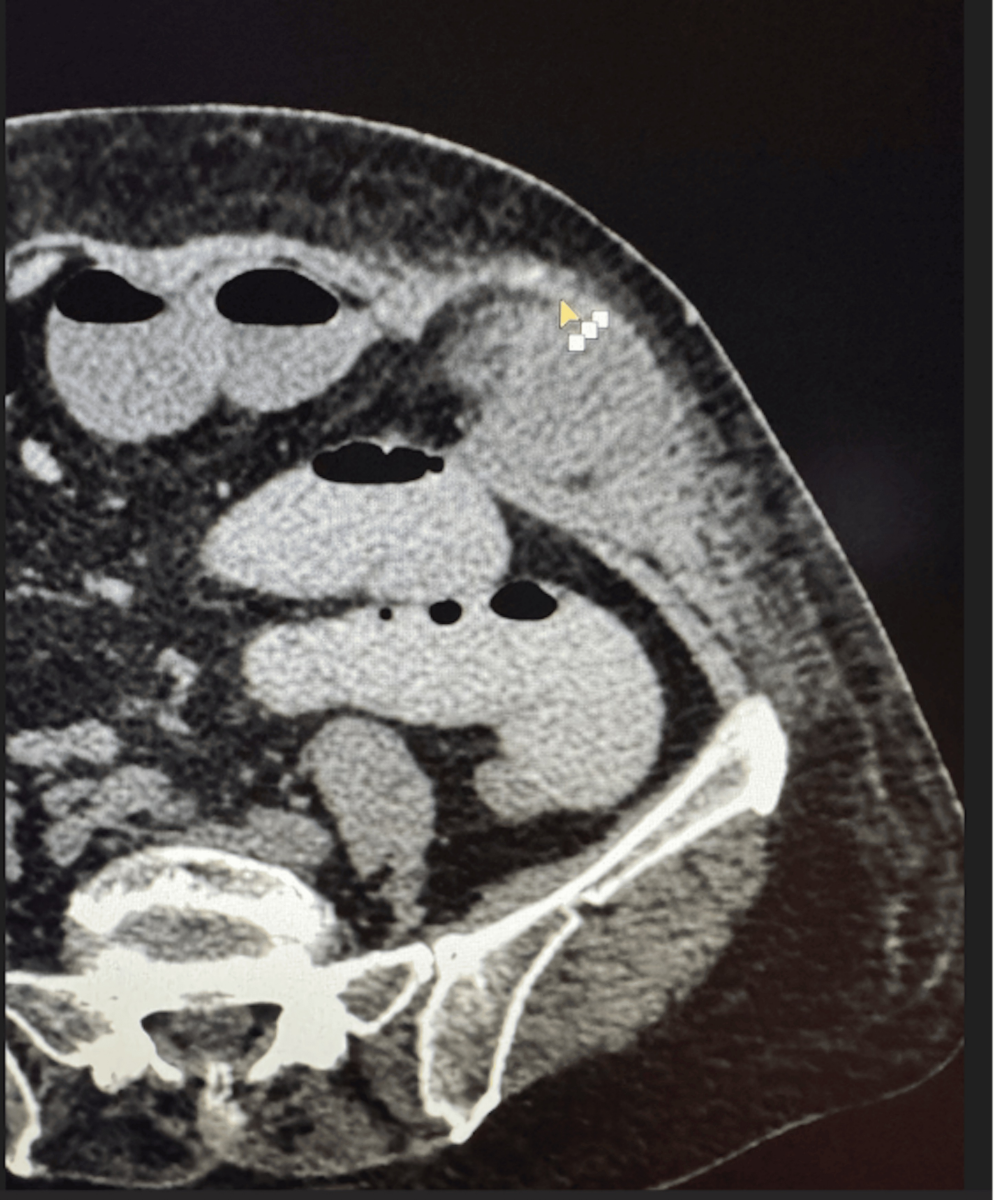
Abdominal CT with oral contrast: Spigelian hernia at the left trocar site The arrowhead highlights the placed suture and the intact anterior rectus sheath.
